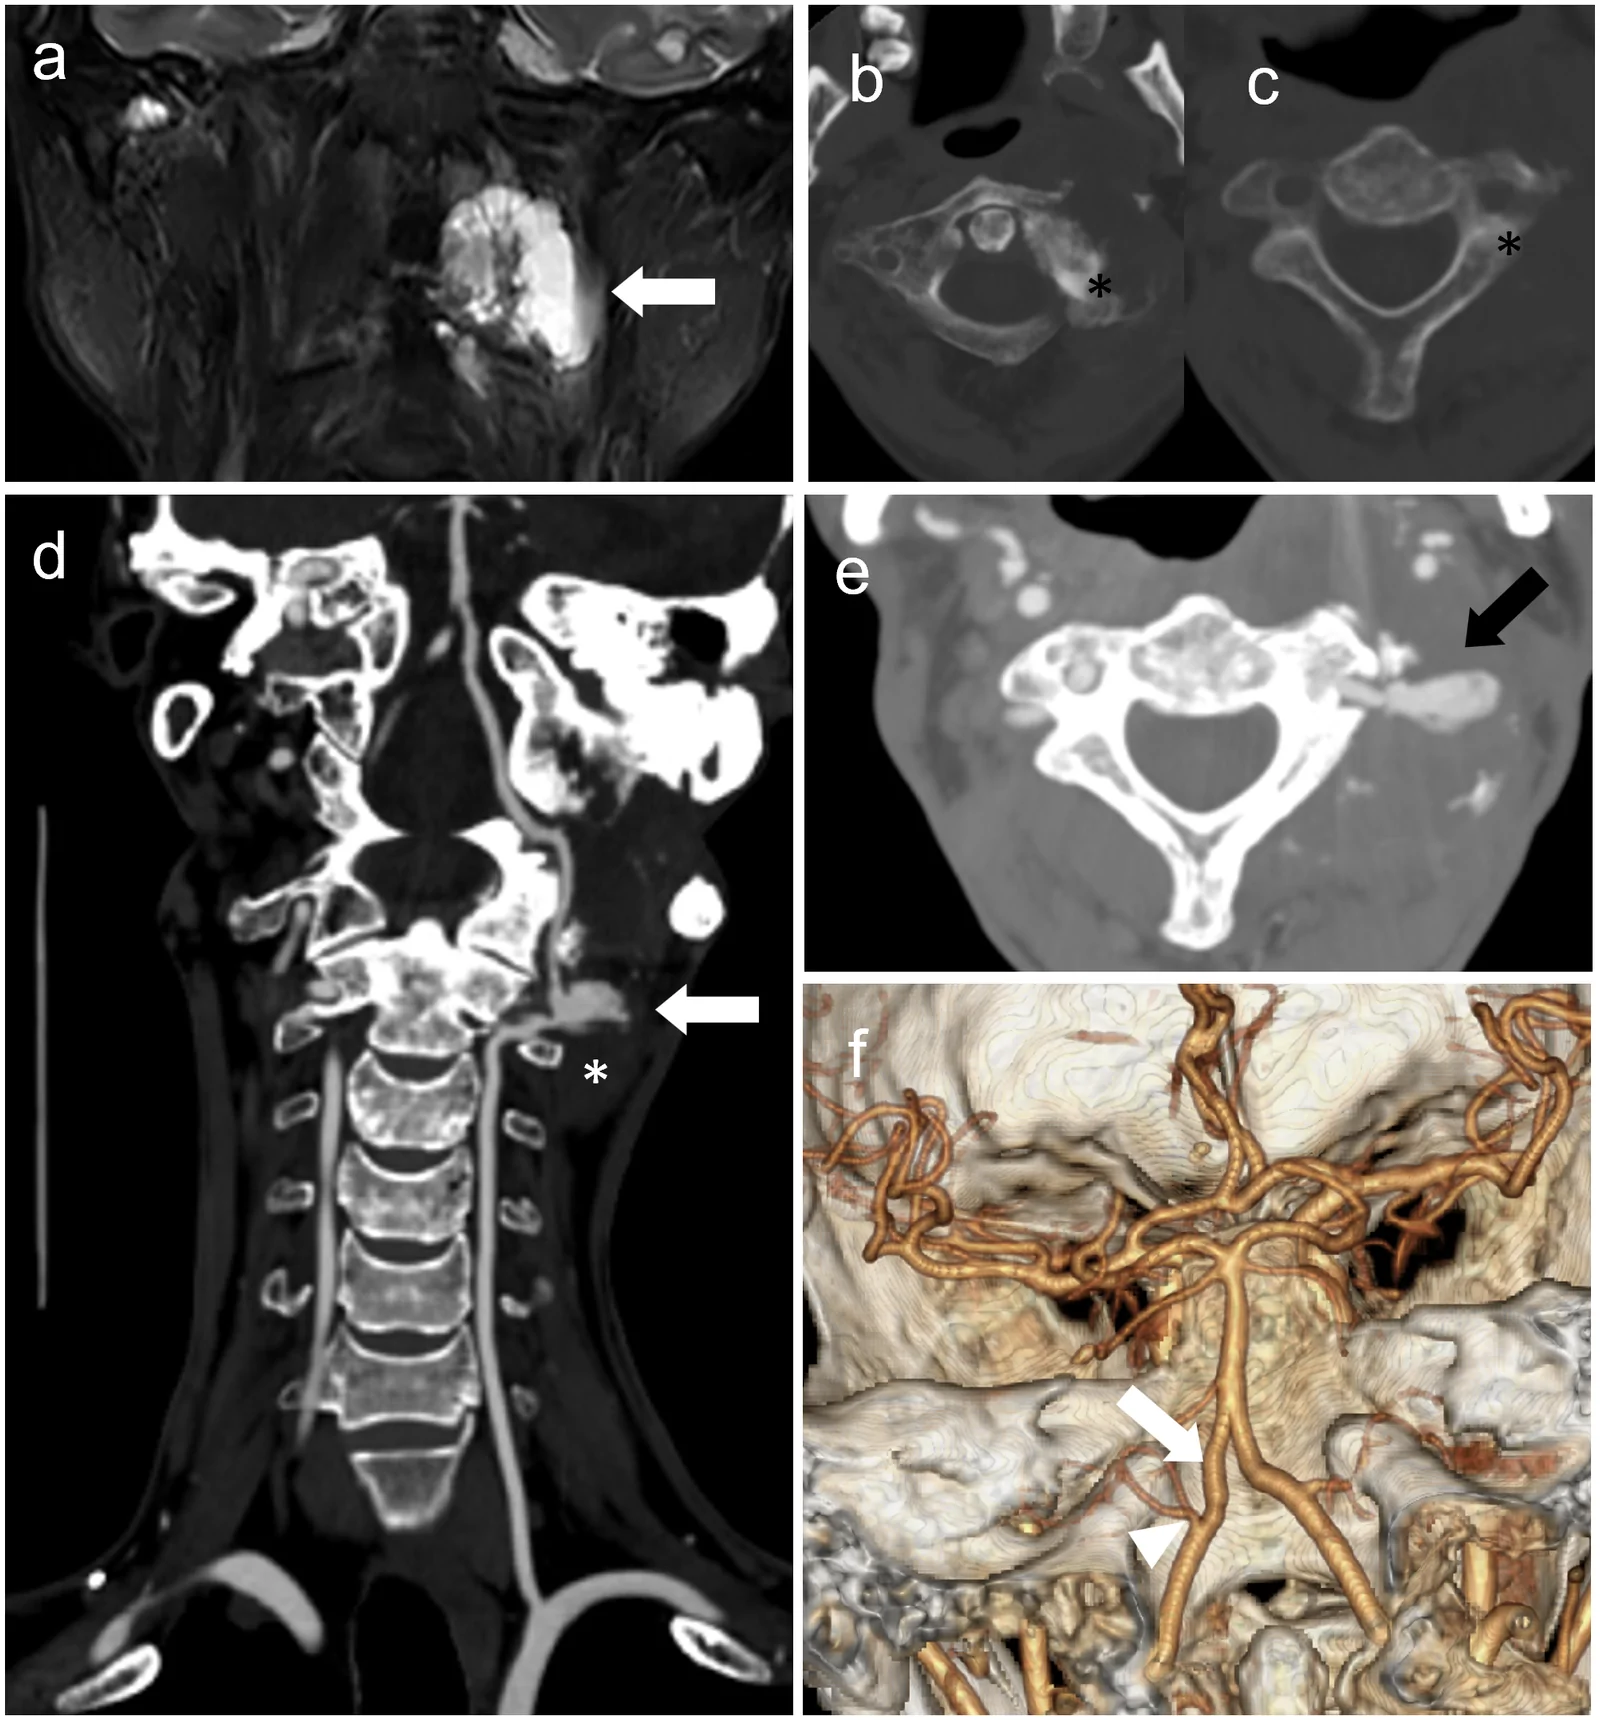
Cervical and vascular imaging after radiotherapy for NPC. (a) Coronal MRI showed a heterogeneous left paravertebral mass involving the upper cervical region (white arrow). (b, c) Axial CT bone-window images showed mixed osteolytic and sclerotic destruction of the left atlas and axis (asterisks). (d) Coronal computed tomography angiography showed the pseudoaneurysm adjacent to the destructive C1–C2 lesion and infiltrative soft-tissue mass (white arrow and asterisk, respectively). (e) Axial computed tomography angiography demonstrated an irregular pseudoaneurysm arising from the left vertebral artery (black arrow). (f) Posterior three-dimensional computed tomography angiography volume rendering showed opacification of the distal left vertebral artery and left posterior inferior cerebellar artery (arrowhead and arrow, respectively).
